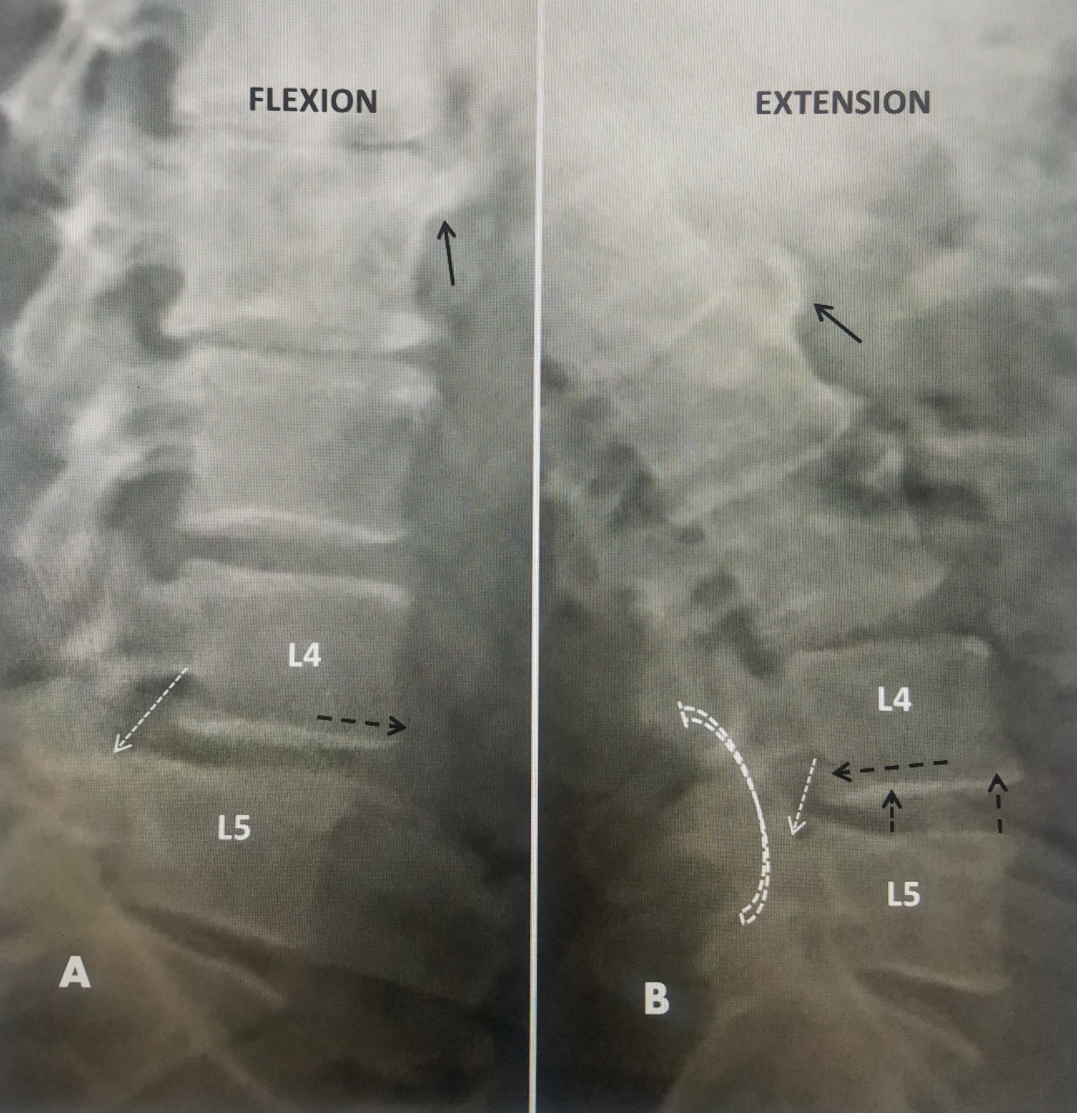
Flexion and extension plain radiographs made to evaluate spinal movement and stability. A: Flexion films show sagittal anterolisthesis at L4-5 (dashed black arrow). The neural foraminal is distorted and mildly reduced in superior-inferior dimension (dashed white arrow). B: Extension films in the same patient shows both posterior rotation (open white curved dashed arrow) and posterior translation of L4 on L5 (dashed black arrow). At the same time the disc space opens anteriorly and narrows posteriorly (solid black arrows). As a result, the neural foramina becomes smaller in extension compared to flexion (solid white arrow) even though the vertebrae are more aligned in extension.
